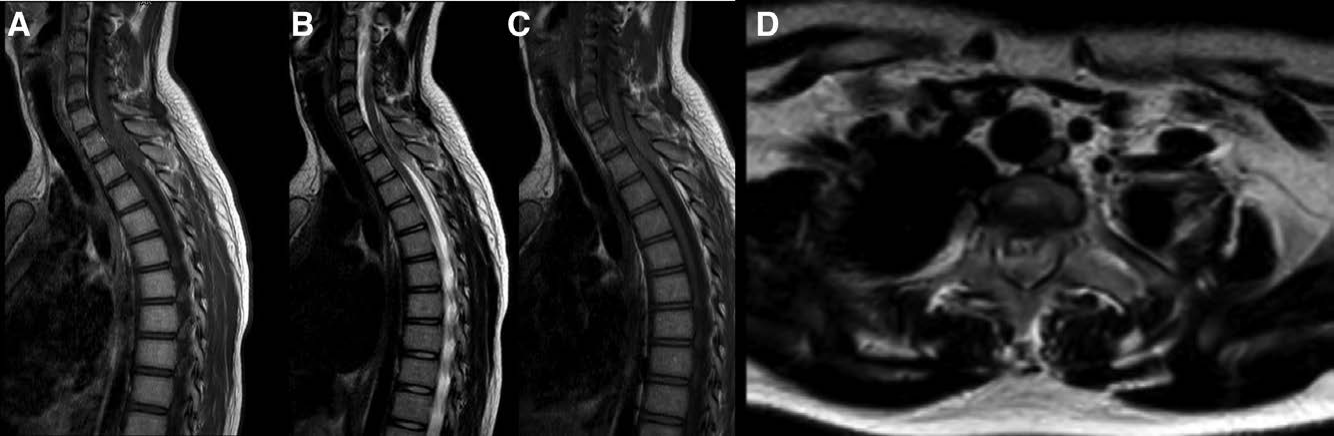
Before the surgery. MRI of the cervical and thoracic spine shows a dumbbell-shaped epidural mass in the posterior aspect compressing the spinal cord at the T1–T2 level. (A) Sagittal T1WI. (B) Sagittal T2WI. (C) Sagittal T1WI with contrast. (D) Axial T2WI. MRI = magnetic resonance imaging.
